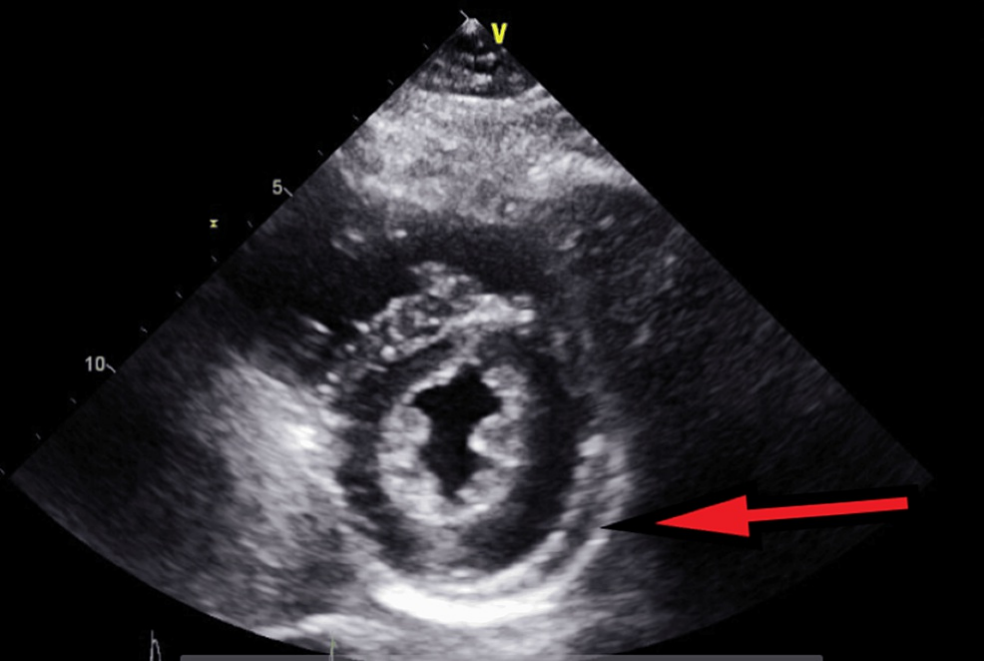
Transthoracic echocardiography - image 2 Transthoracic echocardiography on admission; parasternal short axis view shows a small rim of pericardial fluid collection indicative of the presence of minimal pericardial effusion
